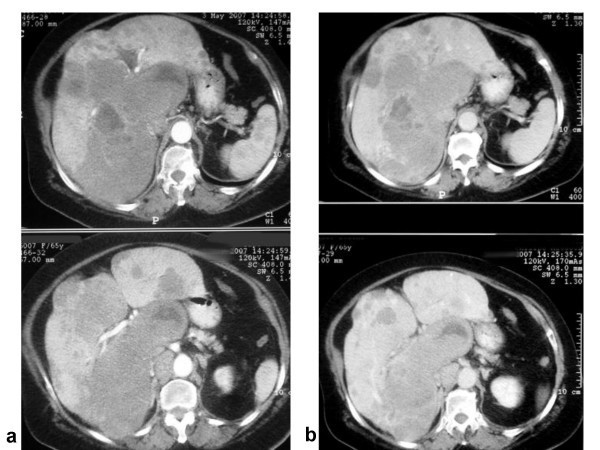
Contrast enhanced CT, arterial (a) and portal venous phases (b): many lesions are demonstrated in both liver lobes showing progressive contrast enhancement.
